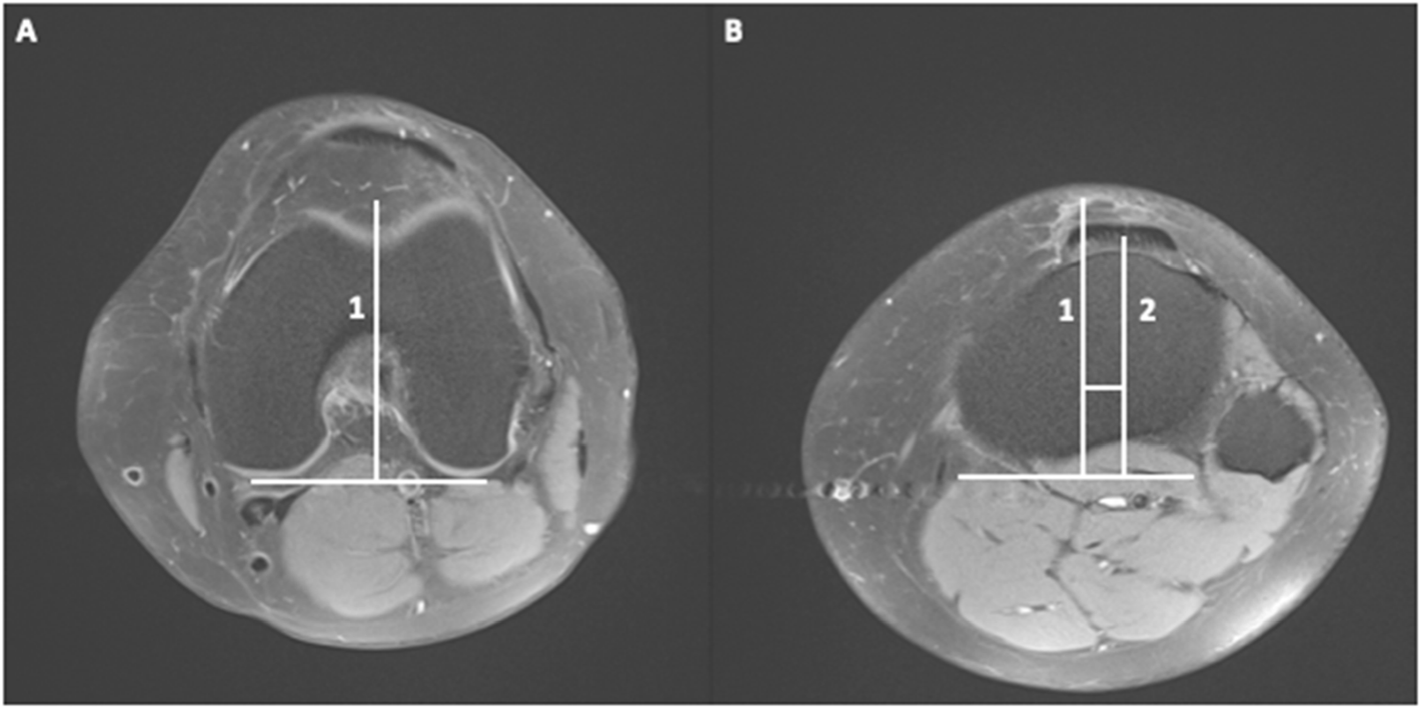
PD MR images of a 34-year-old healthy male subject. a Line 1 was drawn through the deepest cartilaginous point of the trochlear groove to form a 90° angle with the posterior condylar line. b Line 2 was drawn in parallel to line 1 from the center of the patellar tendon (closest to the tibial tubercle) extending to the original posterior condylar line. The distance between lines 1 and 2 represents the TT-TG
